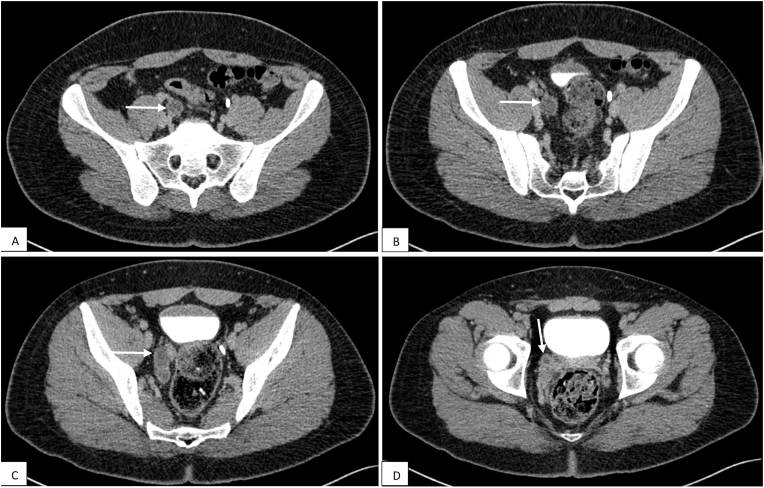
(A–C) Axial CT urogram at 8 minute post intravenous contrast injection shows a blind-ended dilated right ureter (arrows in A-C). The distal ureter inserts ectopically into the right seminal vesicle (arrow in D).
